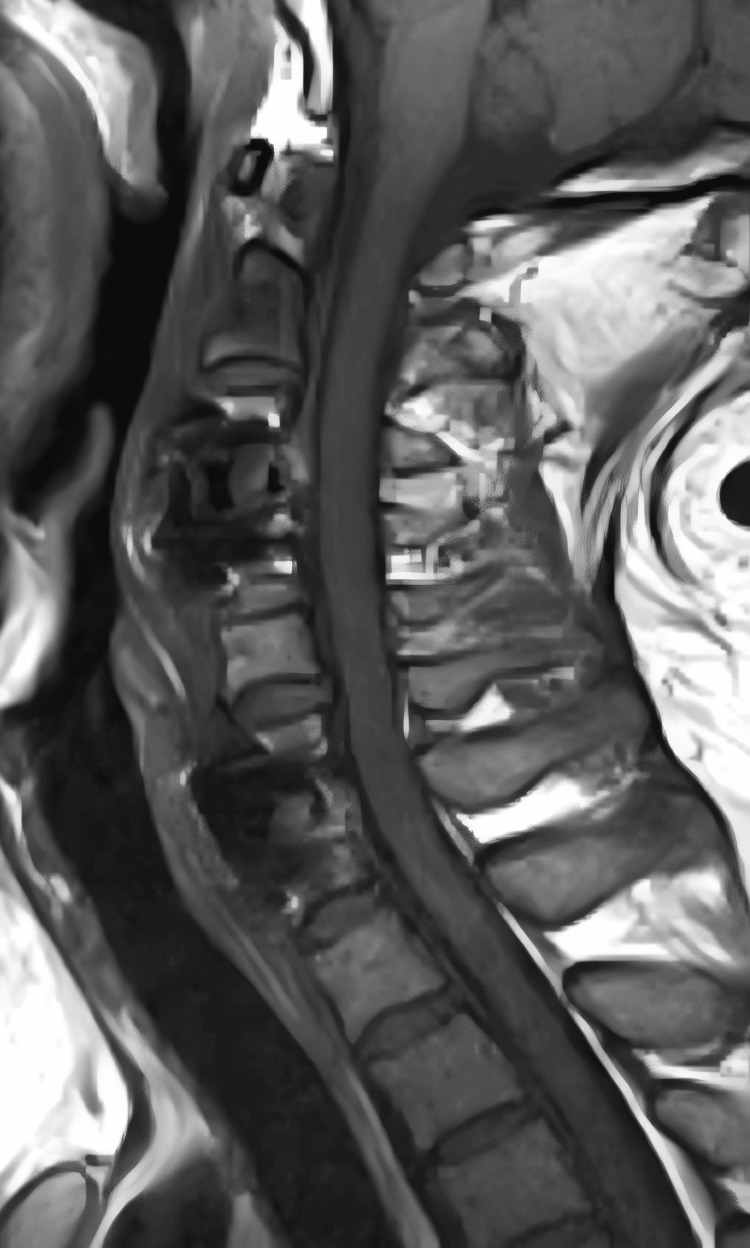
Postoperative MRI Postoperative cervical MRI shows that the hematoma has been completely removed.
